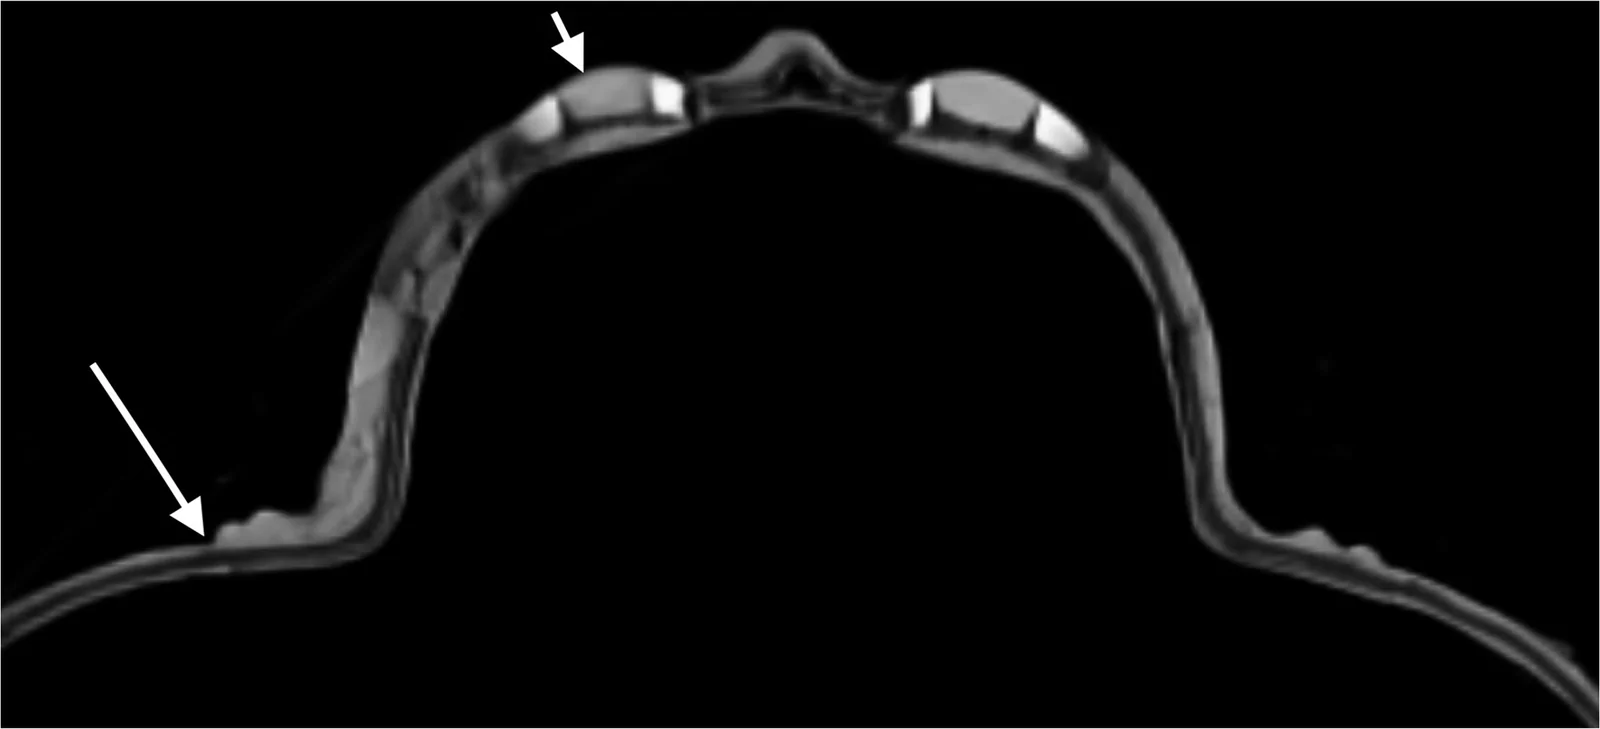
Axial CT image of the face mask at the level of the eyes. The face mask is mounted on the cartonnage (long arrow); the cartonnage is formed of a central low-density layer of linen/papyrus covered by denser layers of gesso (long arrow). Each eye of the mask is made of inlaid stones of a central discoid structure that corresponds to the black obsidian pupil and denser pieces on each side for the white of the eye likely made from quartz.
